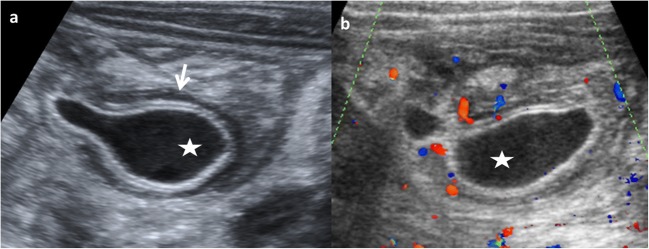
An 8-month-old boy baby with continuous crying is taken to the emergency department. Because of high suspicion of intussusception, a US exam is required. a Abdominal pear-shaped cystic lesion (*star*) in the left flank was found in a coronal US view. It shows a typical outer hypoechoic wall and inner hyperechoic layer, with hypoechoic content inside. b Doppler US showing light hyperaemia of the lesion wall that was also thickened. Surgical findings: complicated duplication cyst in the colonic splenic flexure
